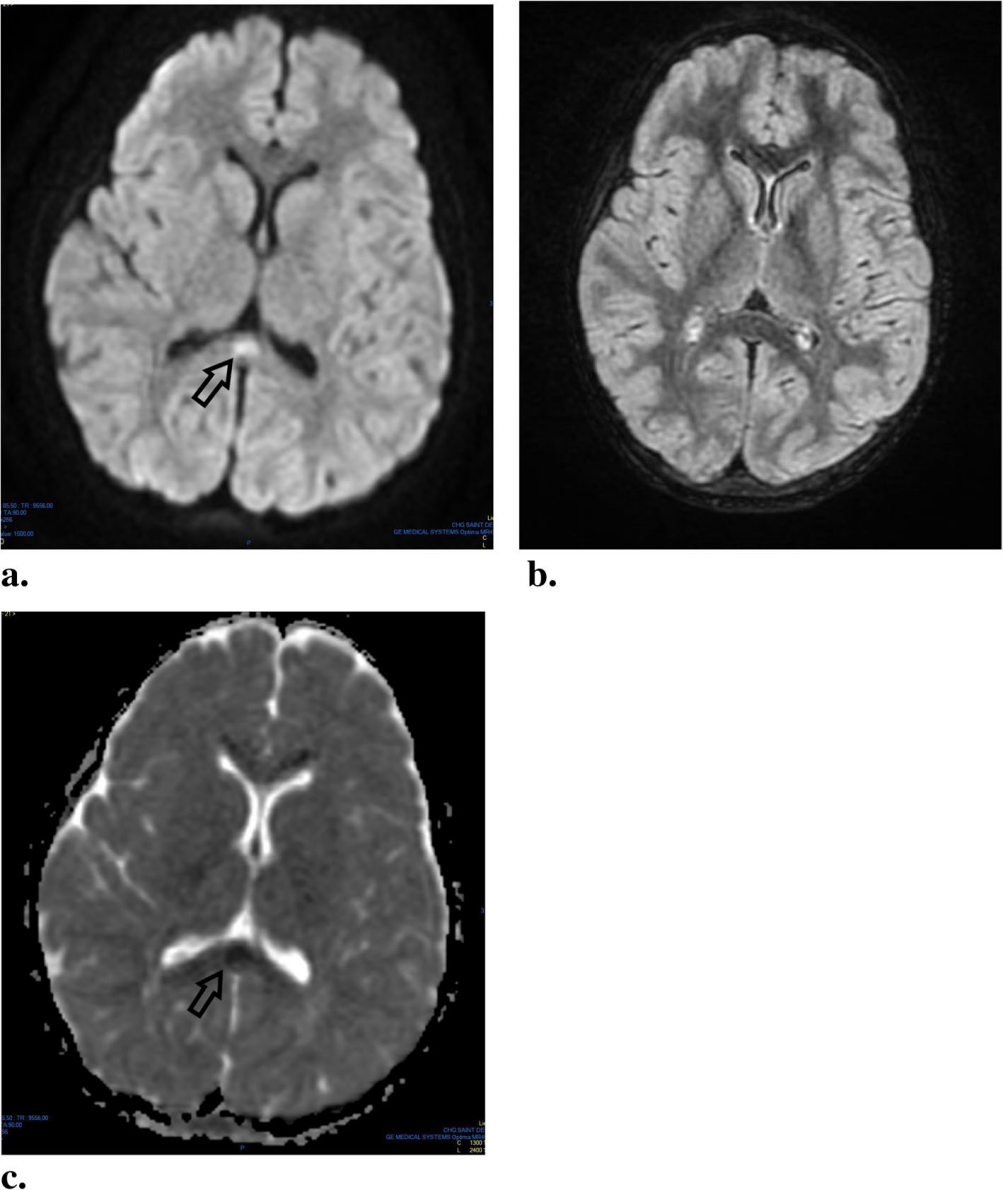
This is the legend from a figure from the study. a. Brain MRI performed on day 2 of the symptoms. Hyperintense signal changes of the SCC are visible on Diffusion-weighted imaging sequence. b. Brain MRI performed on day 2 of the symptoms. No hyperintense signal changes of the SCC are visible on T2W/FLAIR image. c. Brain MRI performed on day 2 of the symptoms. Reduction of ADC is visible on ADC maps
